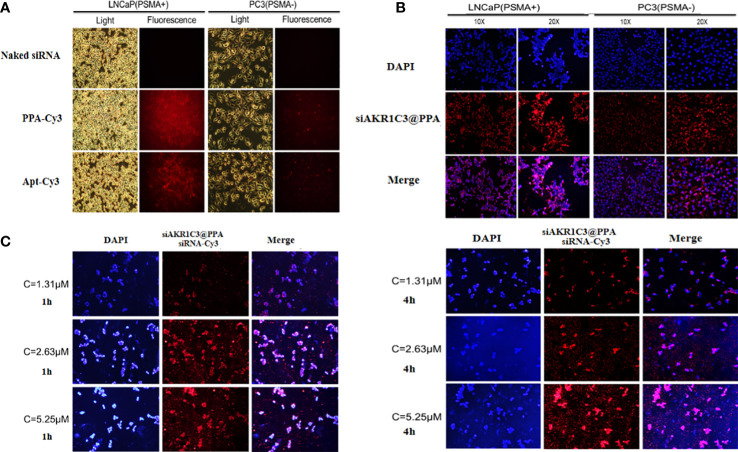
siAKR1C3@PPA can target prostate cancer cells (PSMA^+^). (A) Localization of PPA and aptamer in LNCaP and PC3 cells for 4 h at 37°C. Cy3-labeled aptamer. (B) Localization of siAKR1C3@PPA in LNCaP and PC3 cells for 4 h at 37°C. Cy3-labeled siRNA. (C) The degree of siAKR1C3@PPA internalization is dose- and time dependent. Cy3-labeled siRNA.
